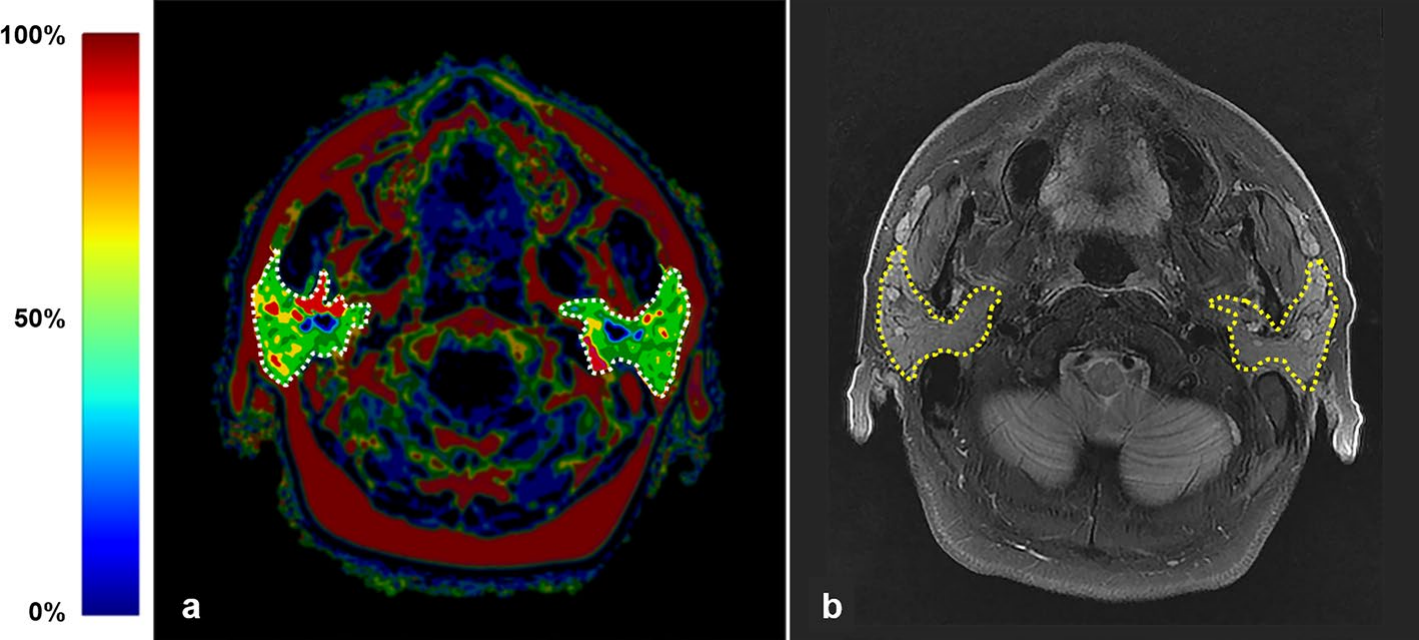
Magnetic resonance imaging presenting the largest axial area of both parotid glands. (a) The fat fraction map image with the selected regions of interest (white dotted line) on the parotid gland. (b) T2-weighted image with fat saturation. Note that the vessels and duct are excluded and only the parenchyma is included. The selected regions of interest are indicated by yellow dotted lines.
